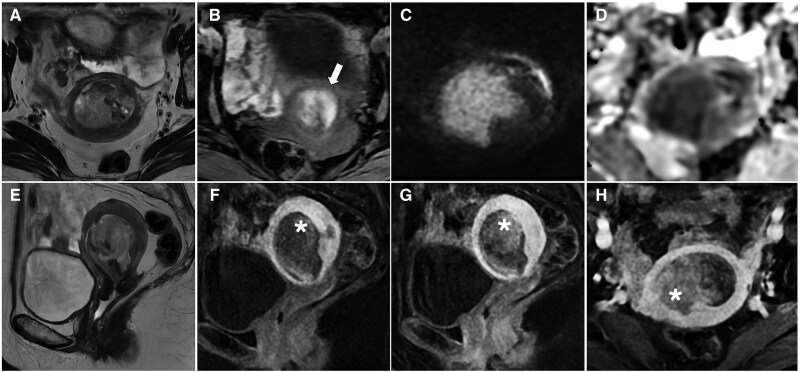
Endometrial carcinosarcoma. MR images show a large endometrial-based lesion extending through the internal os, demonstrating heterogeneous appearance on T2-WI (A, E) and T1-WI (B) due to the presence of haemorrhagic areas (arrow), and marked impeded diffusion (C, D). Arterial (F), venous (G), and delayed (H) postcontrast images show progressive and persistent enhancement (asterisk).
